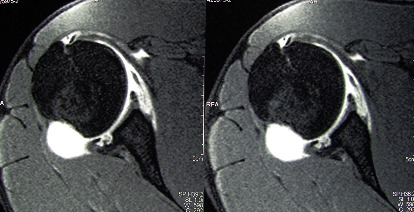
FS-PD-TSE MRI sequences depicting posterior labrum avulsion and posterior chondral lesion with a fatigue fracture of the subchondral bone and degenerative cyst.
